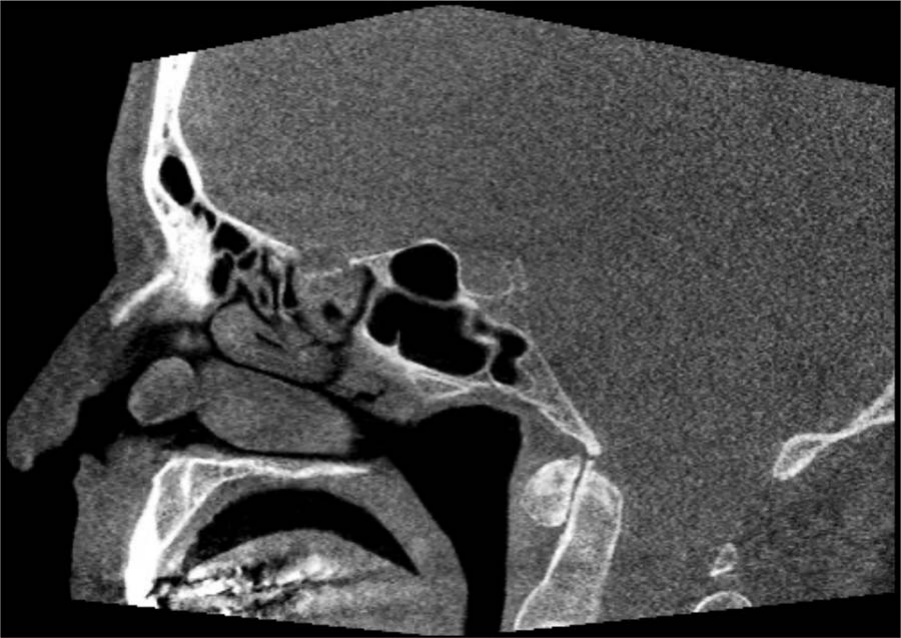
Sagittal non-contrast CT scan images of soft tissue lesion on right inferior turbinate.
